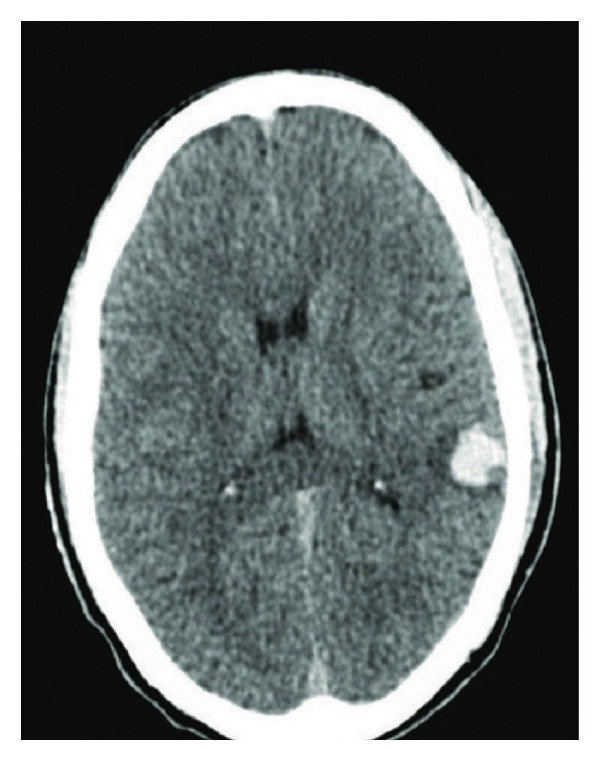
Noncontrast CT scan of the brain showing acute intracerebral hemorrhage in the left temporoparietal region.
